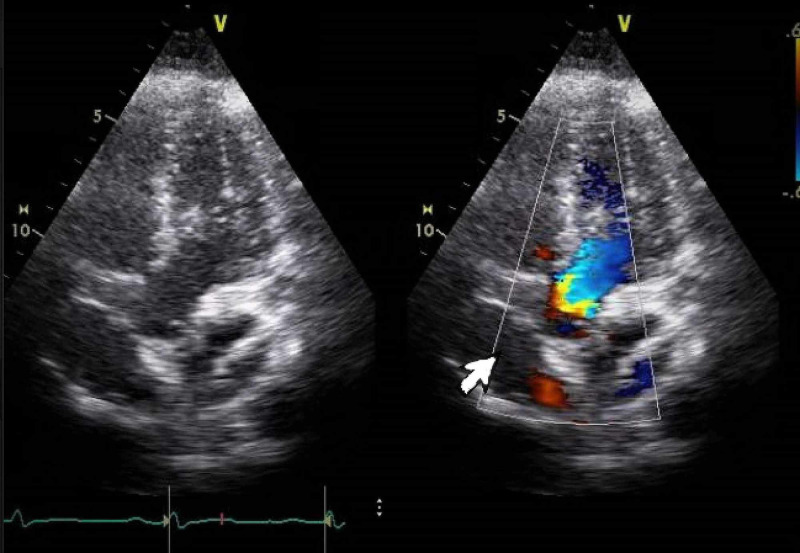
Transthoracic echocardiography with colored Doppler flow: white arrow shows patent foramen ovale.
